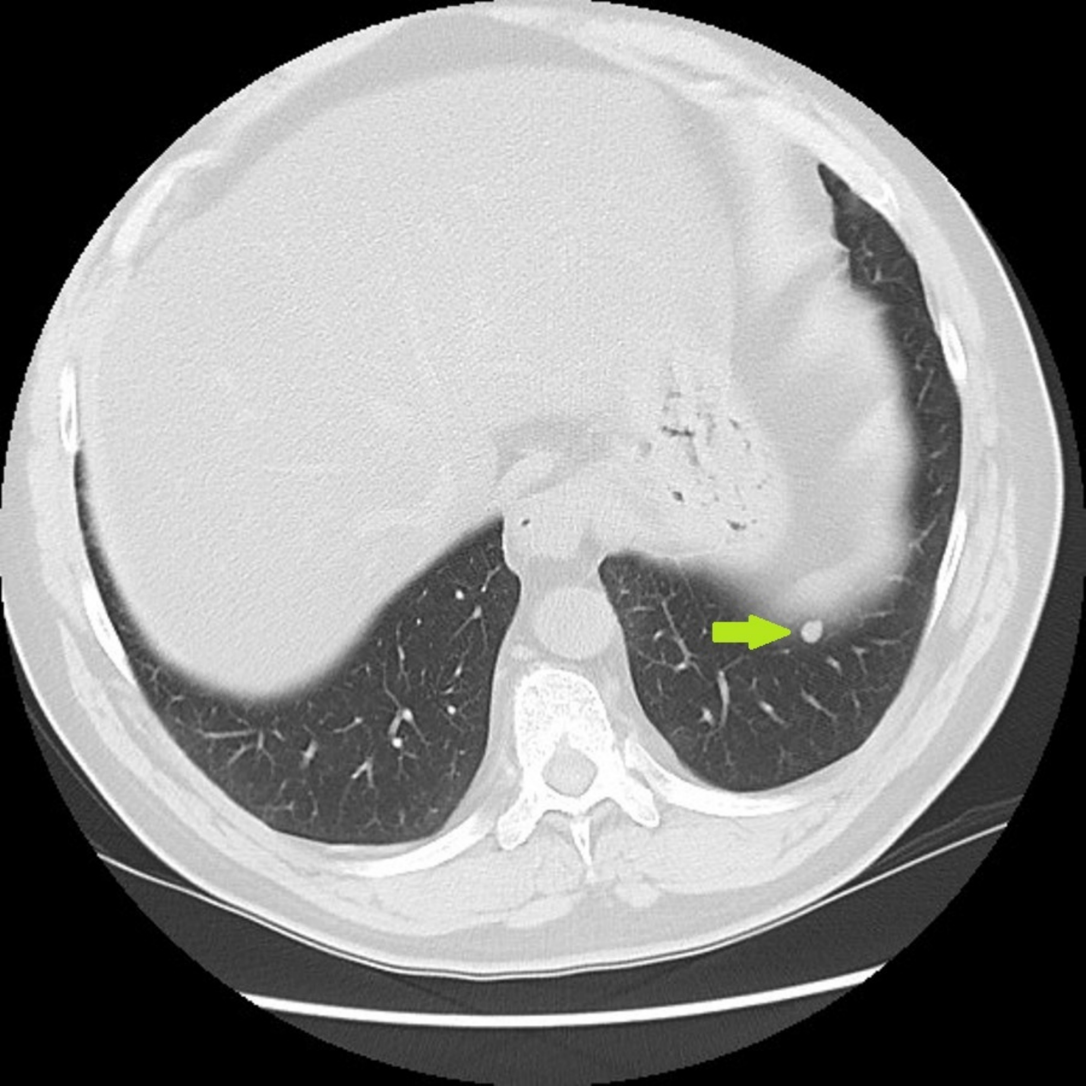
10/2012: One year after resection of primary tumor, computed tomography (CT) lung shows new left lower lung nodule.
